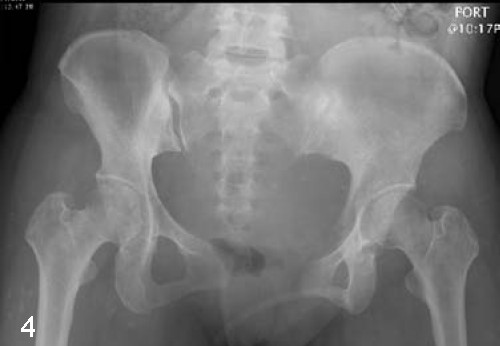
X-rays showing the symphysis pubis with 4.0 cm diastasis initially.
